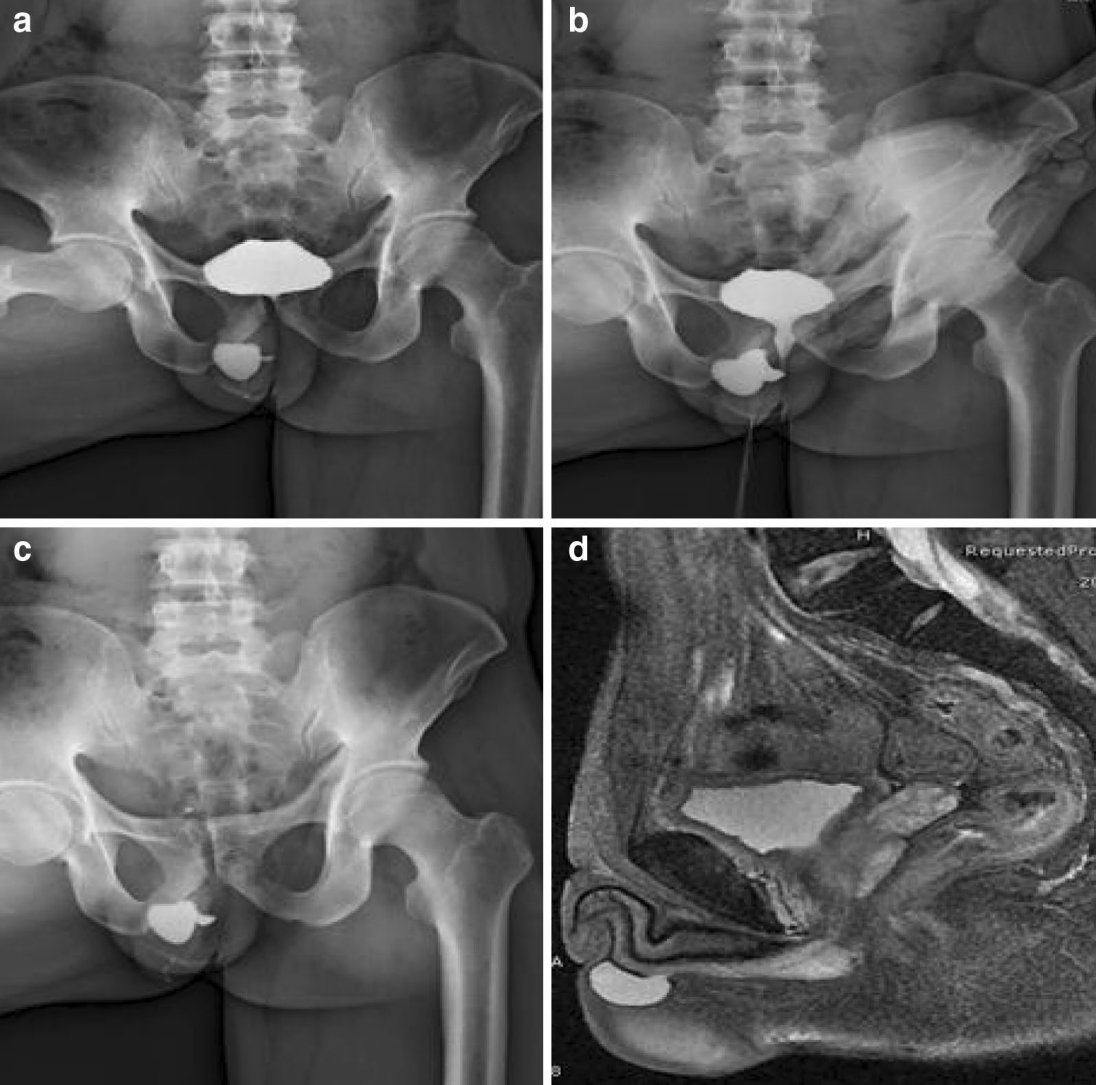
a Retrograde urethrogram showing the urethral diverticulum at the penoscrotal level (*A full bladder period*). b Retrograde urethrogram showing the urethral diverticulum at the penoscrotal level (*Emptying period*). c Retrograde urethrogram showing the urethral diverticulum at the penoscrotal level (*Empty period*). The swelling is still remain contrast filled after urethral emptying. d MRI reveals a fluid and hypodense mass correlated with urethra
